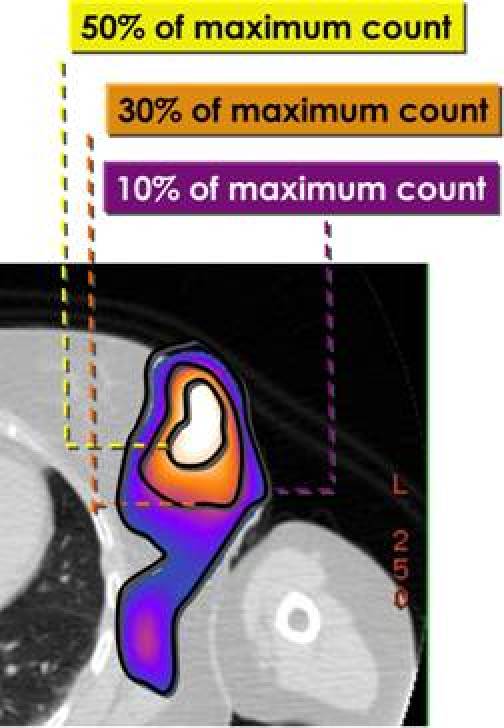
a: SPECT/CT fused images showing a hot region in the operated left breast. b: The hot region was divided into three different areas: high-uptake area (uptake higher than 50% of the maximum—isorois 50%); medium-uptake area (between 50% and 30% isorois) and low-uptake area (between 30% and 10% isorois).
